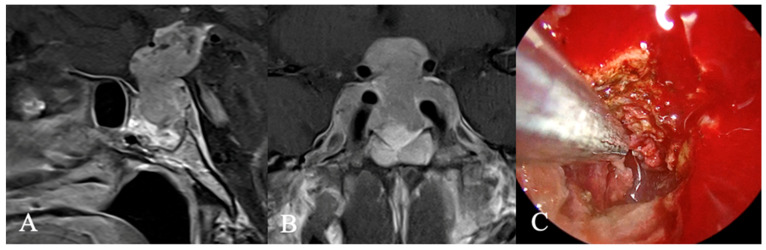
Metastatic tumor lung cancer of a 74-year-old male patient. (A,B) Sagittal and coronal contrast-enhanced MRI reveal an intrasellar and suprasellar tumor infiltrating the surrounding tissue. (C) Intraoperative findings confirmed the presence of firm, tightly adherent tissue with a high risk of collateral injury. The well-vascularized tumor invaded both parasellar and suprasellar structures, making gross total resection rarely feasible due to the risk of vascular damage.
